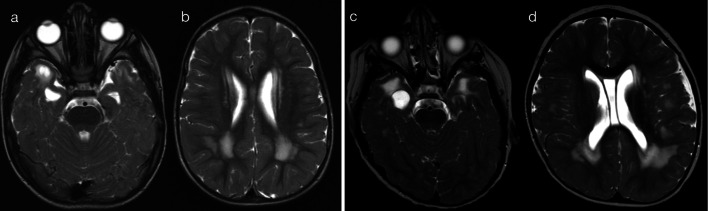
T2 hyperintensity in supratentorial white matter—without neuronal migration abnormality (Pattern 4B). Case 1: 2-year-old child with congenital left sensorineural hearing loss. Guthrie test was positive for CMV. T2-weighted imaging (a, b) demonstrated cystic change at the right anterior temporal pole, with bilateral parietal and periventricular white matter hyperintensity. Case 2: 2-year-old child with developmental delay. Guthrie test was positive for CMV. T2-weighted imaging (c, d) demonstrated cystic change at the right anterior temporal pole, with bilateral peritrigonal white matter hyperintensity
